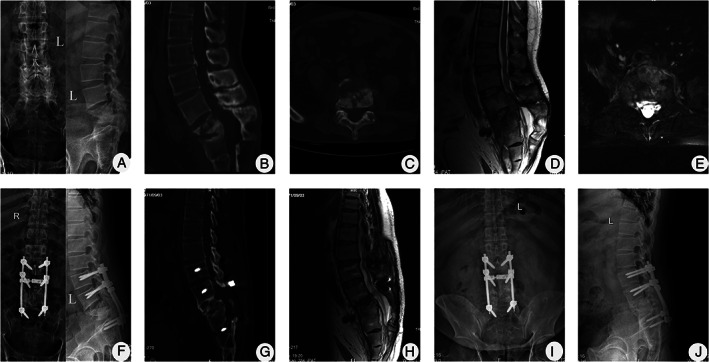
A 39‐year‐old male with L_5_/S_1_ TB underwent one‐stage posterior debridement, interbody fusion, and lumbosacral fixation. (A) Anterior and lateral radiographs of the lumbar spine showing the affected vertebrae with a lumbosacral angle of 22°. (B–E) Preoperative CT and MRI demonstrating that the L_5_ vertebral body was severely damaged and collapsed, with cold abscess formation in the spinal canal. (F) Postoperative X‐ray showing good internal fixation position with a lumbosacral angle of 28°. (G, H) Postoperative CT and MRI showing satisfactory bone fusion at 15 months and an unobstructed spinal canal. (I, J) X‐ray displaying solid bone fusion and no displacement of instruments, with the correction loss of 1° throughout 63 months of follow‐up.
